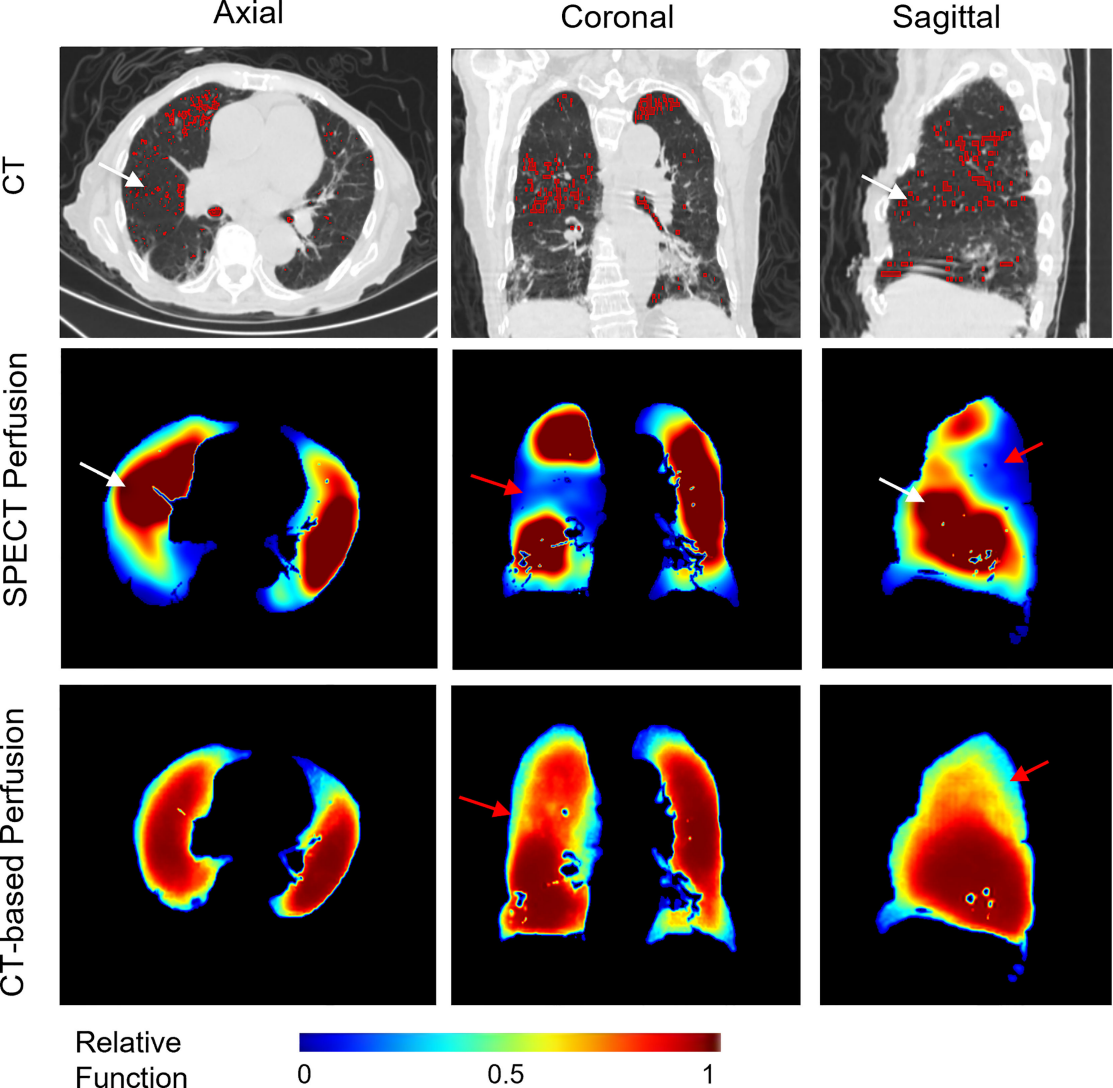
A representative case with relatively low perfusion prediction on CT-based perfusion images. The red contours in the CT images indicate the low intensity regions (<-900 HU). The red arrows indicate the main mismatched low functional regions. The white arrows indicate the inconsistent regions between the low intensity regions of the CT images and the SPECT perfusion images.
